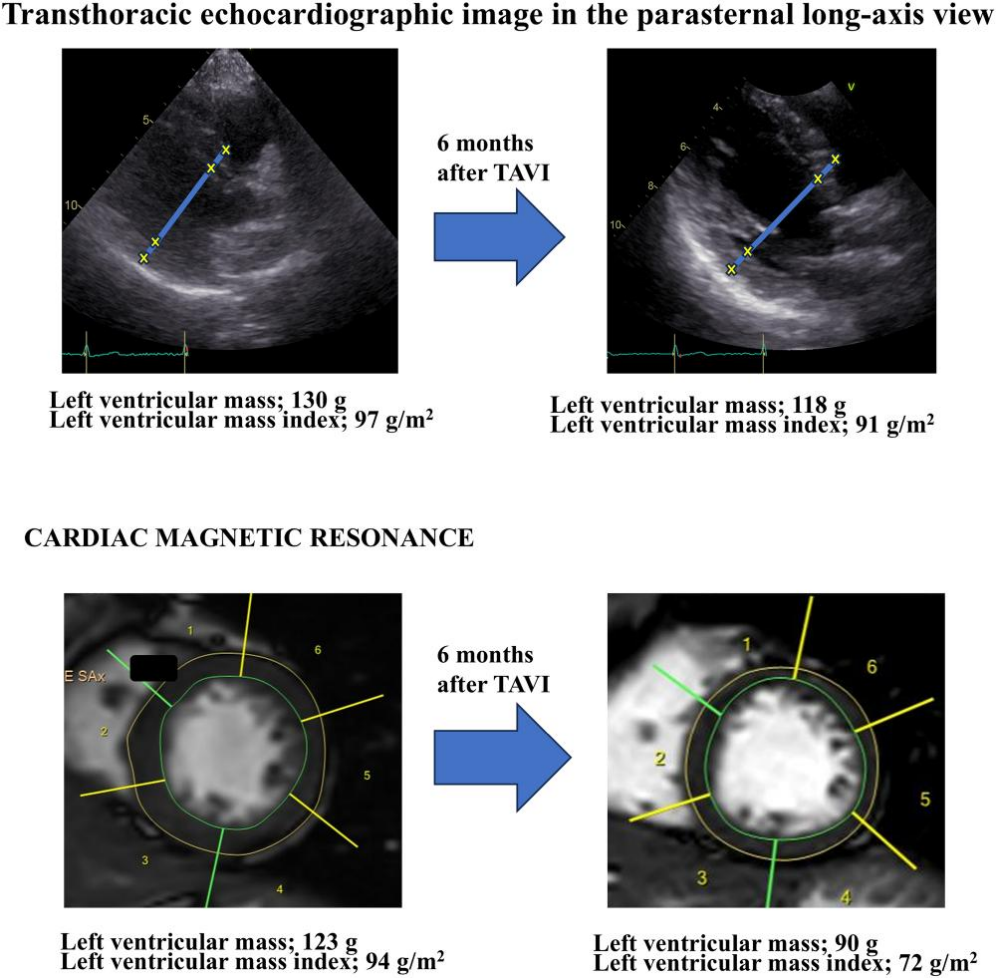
Left ventricular reverse remodelling assessed by echocardiography and cardiac magnetic resonance imaging. Structural changes in the left ventricle before and after transcatheter aortic valve implantation were assessed using transthoracic echocardiography and cardiac magnetic resonance imaging. Echocardiography: the left ventricular mass has decreased from 130 to 118 g, and the left ventricular mass index has decreased from 97 to 91 g/m². The interventricular septal thickness has also decreased from 11 to 8 mm. Cardiac magnetic resonance imaging: the left ventricular mass has decreased from 123 to 90 g, and the left ventricular mass index has decreased from 94 to 72 g/m². These findings indicate significant left ventricular reverse remodelling following relief from pressure overload after transcatheter aortic valve implantation.
